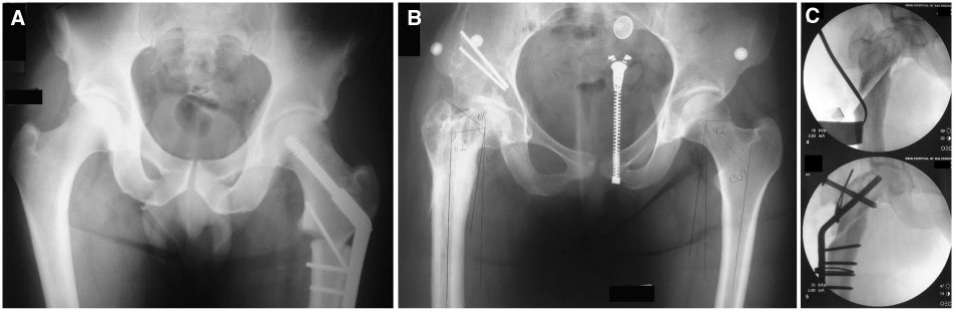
With a subtrochanteric valgus osteotomy (modified Wagner-type), the greater trochanter (GT) is essentially advanced distally and laterally. Note the intentional translation at the site of osteotomy (A). Anteroposterior pelvic radiograph of a 19-year-old woman with a history of multiple previous operations for a right congenitally short femur. The GT abuts the pelvis, despite a previous GT transfer. The neck-shaft angle measures 112° (B). The patient underwent a Morscher osteotomy (intra-operative radiographs shown) which involves sliding the femoral shaft distally and laterally along an osteotomy made at the desired neck-shaft angle. The GT is osteotomized and advanced at the same angle (C). Images provided by courtesy of Dror Paley, MD, FRCS(C).
